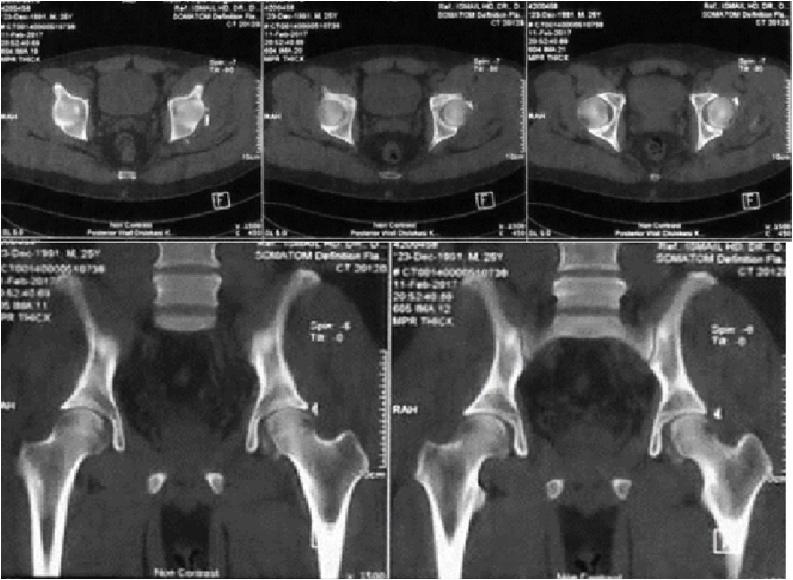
CT Scan: Evaluation of Fragment Size & Location.
